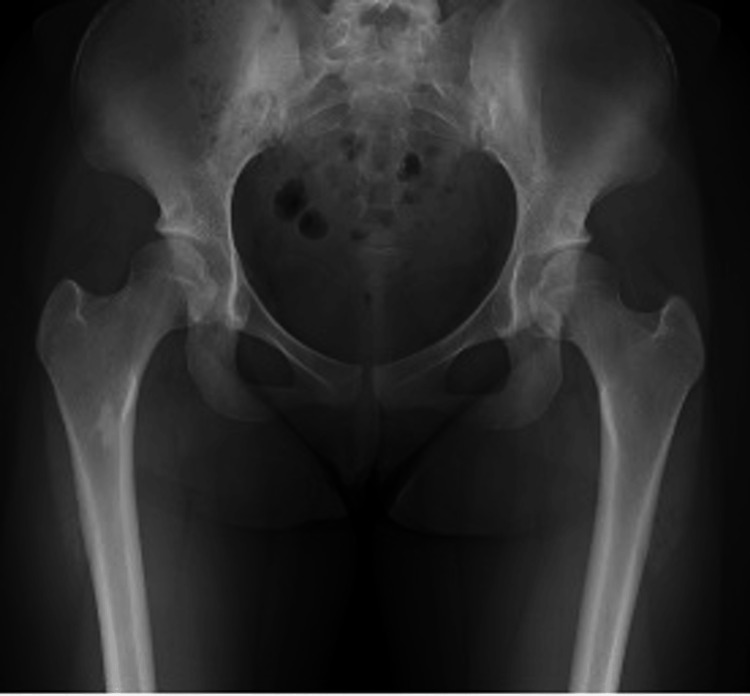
Preoperative radiographs of case 2. CE angle: right 11.6°, left 9.3°; ARO: right 13.7°, left 17.2°; Sharp's angle: right 48.5°, left 48.8°; AHI: right 72.5%, left 71.2%. CE, center–edge; ARO, acetabular roof obliquity; AHI, acetabular head index
